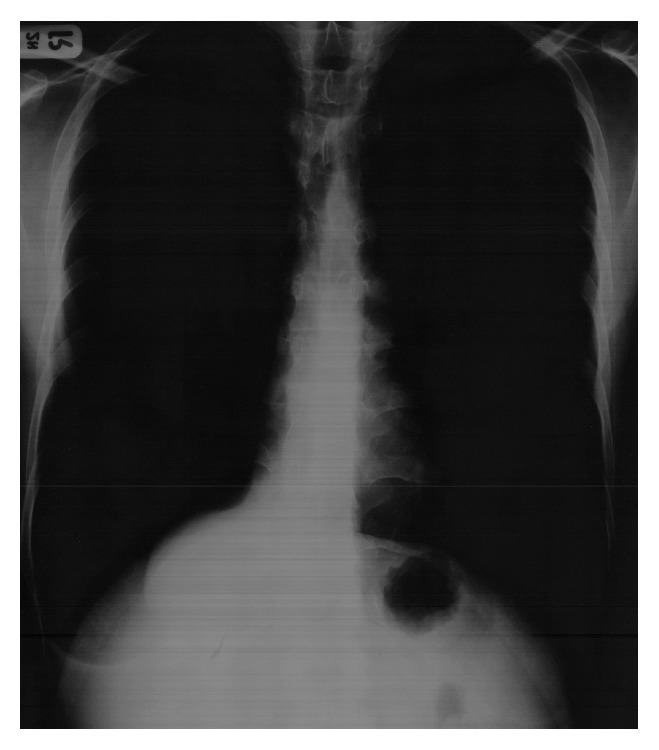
32-year-old man, diagnosed for ectopic kidney; chest X-ray demonstrated hyperinflation of both lungs, with round soft tissue density in the cardiophrenic angle, which does not silhouette the cardiac shadow.
